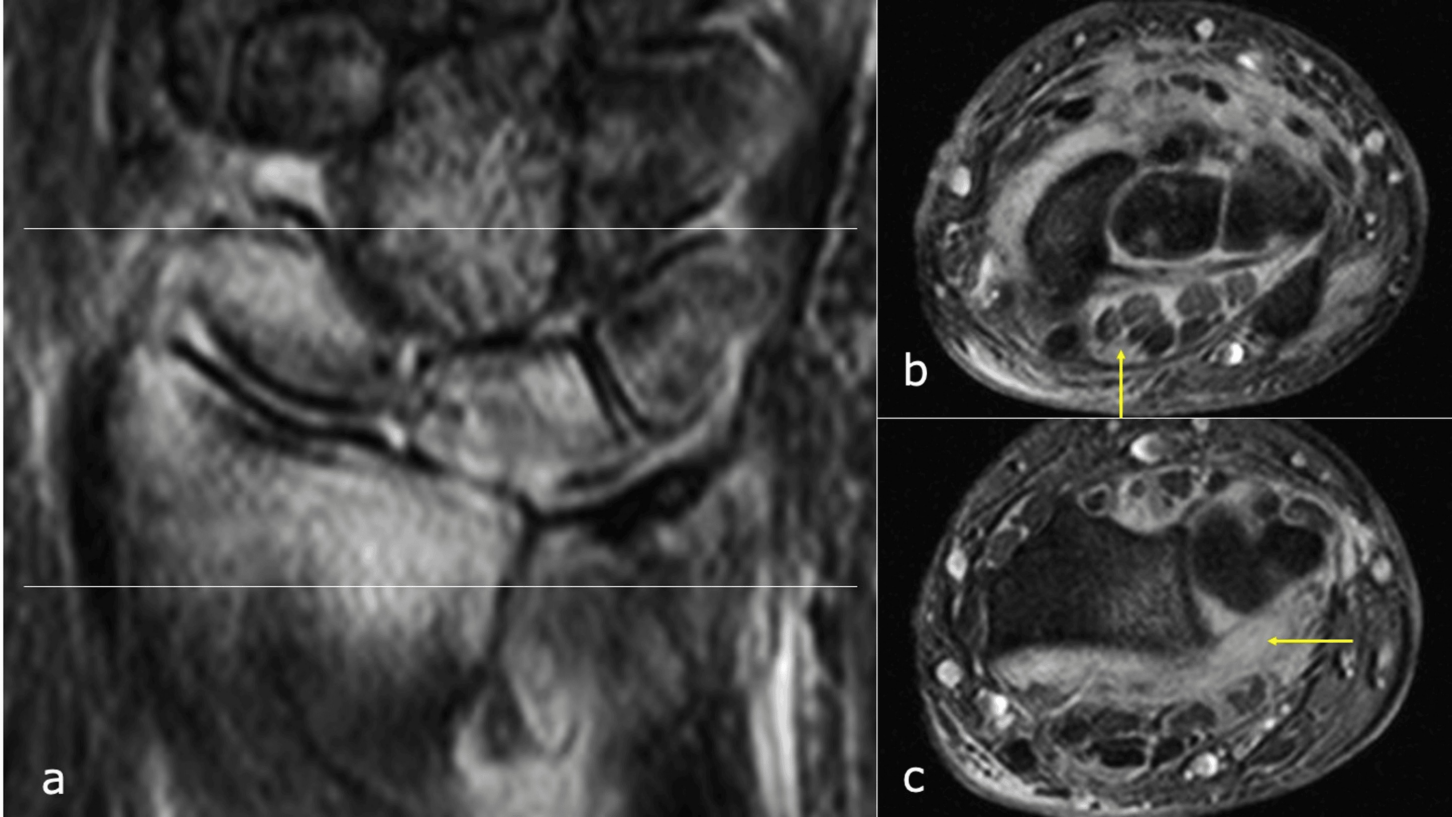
Magnetic resonance imaging three weeks after Kirschner wire removal. a: Coronal view. b: Axial view at the carpal tunnel level. c: Axial view at the distal radioulnar joint level. The inflammatory changes extending to the palmar aspect of the wrist joint and surrounding flexor tendons can be seen (arrow).
